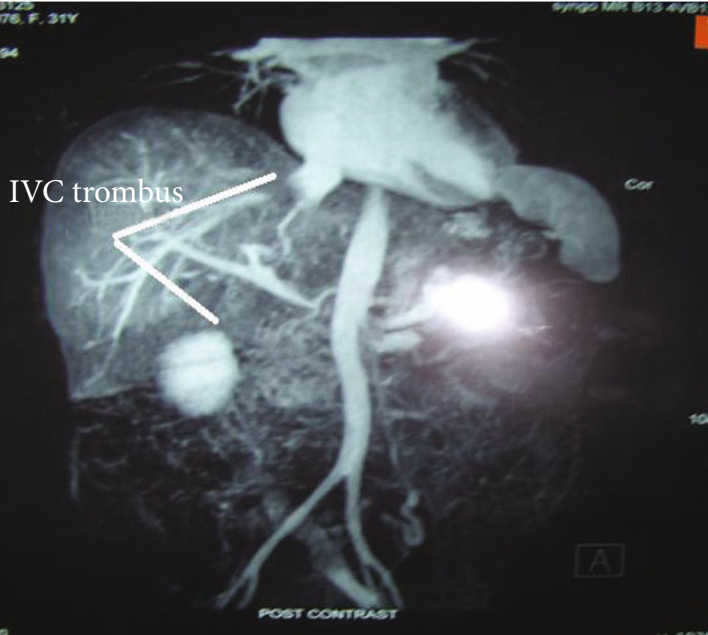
CT of the abdomen showing inferior vena cava defect.
